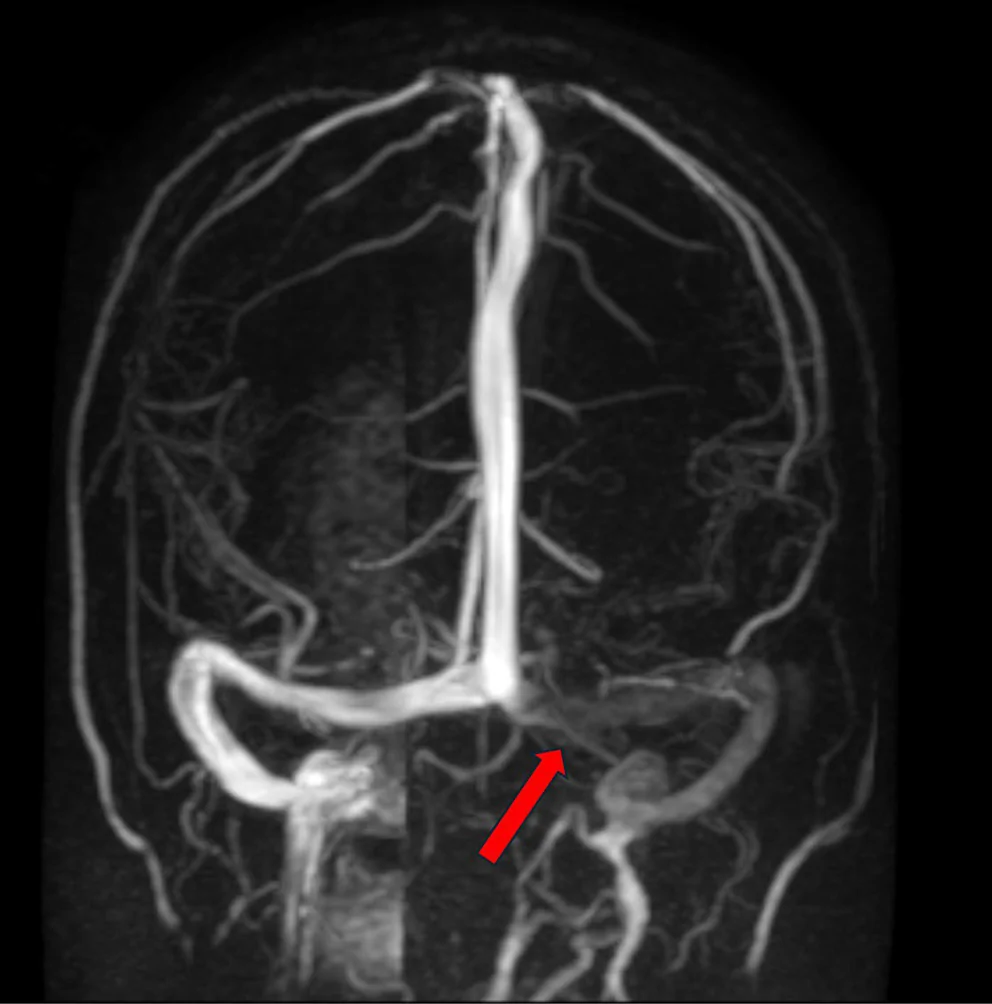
MRI venography image showing right transverse and sigmoid sinus thrombosis (arrow) with no flow in this vein.
